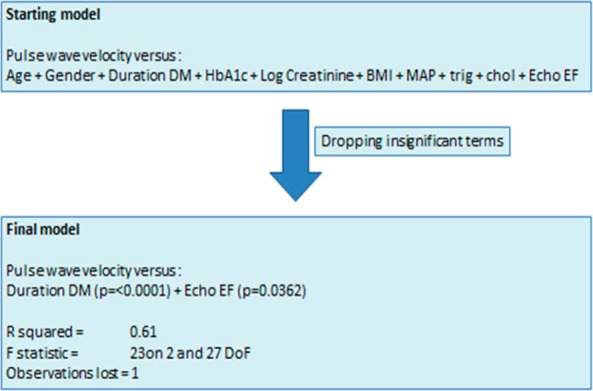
Linear regression model of risk factors associated with arterial PWV in 31 Alström subjects. DM, diabetes mellitus; MAP, mean arterial pressure mmHg; trig, serum triglycerides; Chol, serum non-HDL cholesterol; Echo EF, echocardiogram left ventricular ejection fraction.
